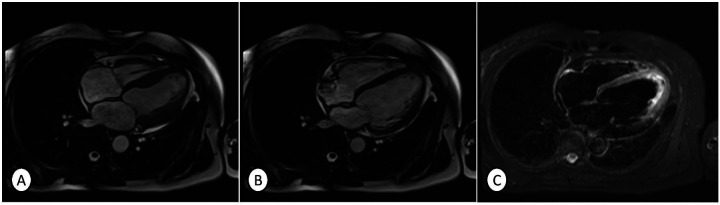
Cardiac magnetic resonance imaging showing a dilated, non-hypertrophic left ventricle with a moderately depressed left ventricular ejection fraction (LVEF 39%) and noticeable hypokinesia in the mid-apical segments during systole (A) and normal contraction in the diastole (B), and T2-STIR sequence showing a marked increase in signal intensity in the mid-apical segments, consistent with diffuse myocardial edema (C). No intracavitary thrombi or pericardial or pleural effusions were observed. Therefore, according to these findings, the patient was diagnosed with TTS.
